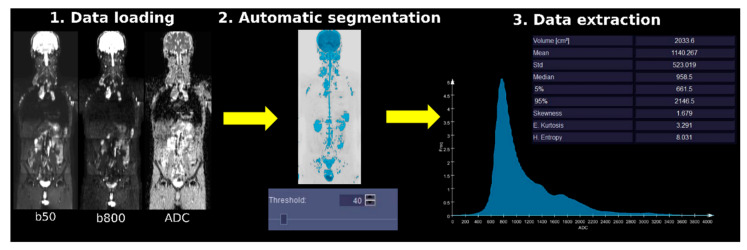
Whole-body diffusion weighted imaging (WB-DWI) image analysis and data extraction process using syngo, via Frontier MR Total Tumor Load software. Reprinted from [56].
